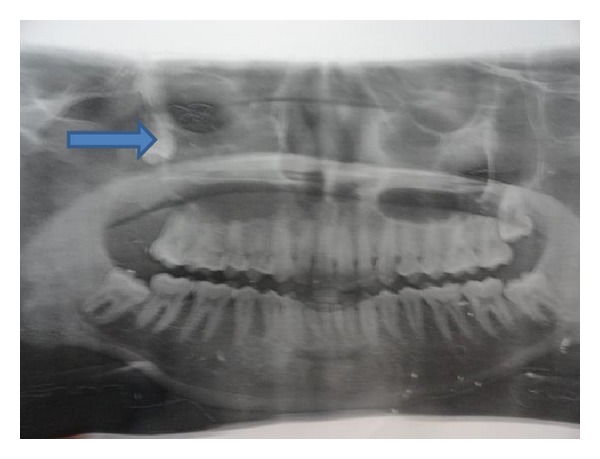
Orthopantomograph showing an ectopic third molar in the right maxillary sinus.
